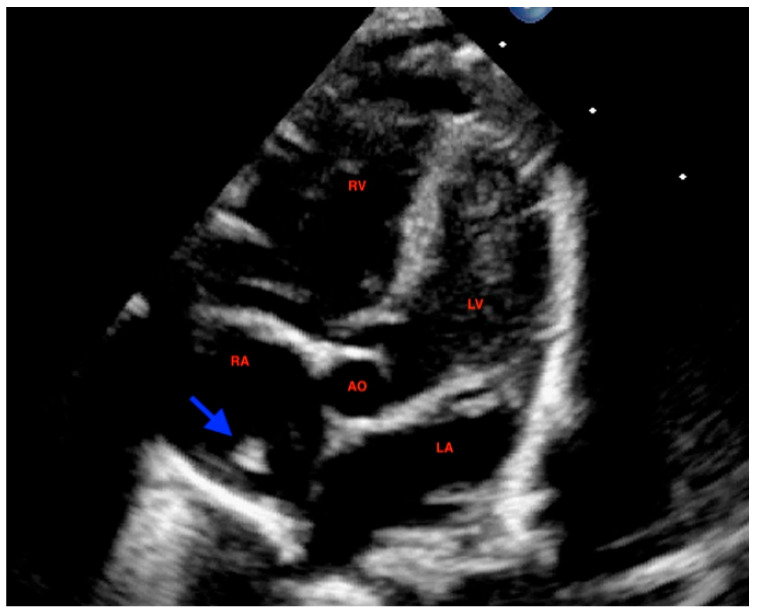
Postanatal apical 5-chamber view: echocardiography at 72 h of age displayed a persistent hyperechoic mass (arrow) within the right atrium compatible with hypertrophic crista terminalis. The structure showed no variations in growth compared to previous scans. RA: right atrium; RV: right ventricle; LA: left atrium; LV: left ventricle; AO: ascending aorta.
